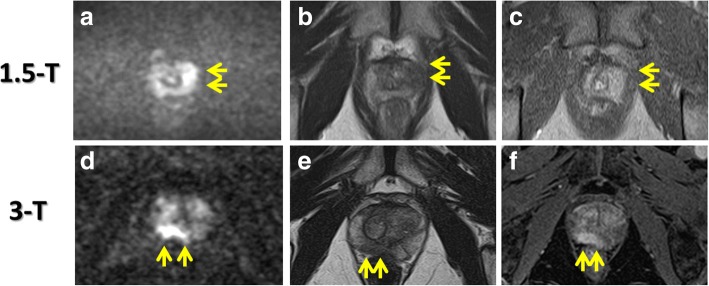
MRI images. 1.5-Tesla MRI images obtained from a patient with a PSA level of 3.86 ng/mL (A − C). Diffusion weighted image (DWI) with a b-value 1500 s/mm^2^ (A) showed a high intensity area in the left peripheral zone (Arrow). T2 weighted image (T2WI: B) and dynamic contrast enhancement image (DCEI: C) showed a low intensity area and an enhancement, respectively, in the same lesion, which was considered to be a high level of suspicious. 3-Tesla MRI images obtained from a patient with a PSA level of 3.57 ng/mL (D − F). DWI with a b-value 2000 s/mm^2^ (D) showed a high intensity area in the right peripheral zone (Arrow). T2WI (E) and dynamic DCEI (F) showed a low intensity area and an enhancement, respectively, in the same lesion, which was considered to be a high level of suspicious
